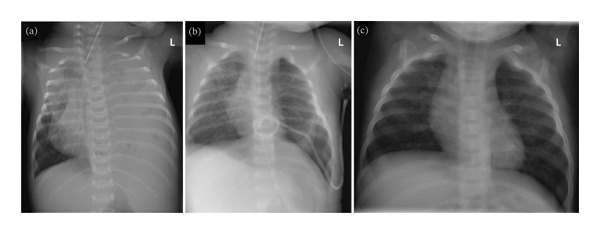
Initial chest radiograph at referring hospital showing a massive left diaphragmatic hernia with herniated bowel loops and rightward mediastinal shift (a). Immediate postoperative image after emergency bedside thoracotomy reveals re‐expansion of the left lung and resolution of mediastinal shift (b). Five‐month follow‐up radiograph shows normal lung aeration and thoracic anatomy without signs of recurrence (c).
